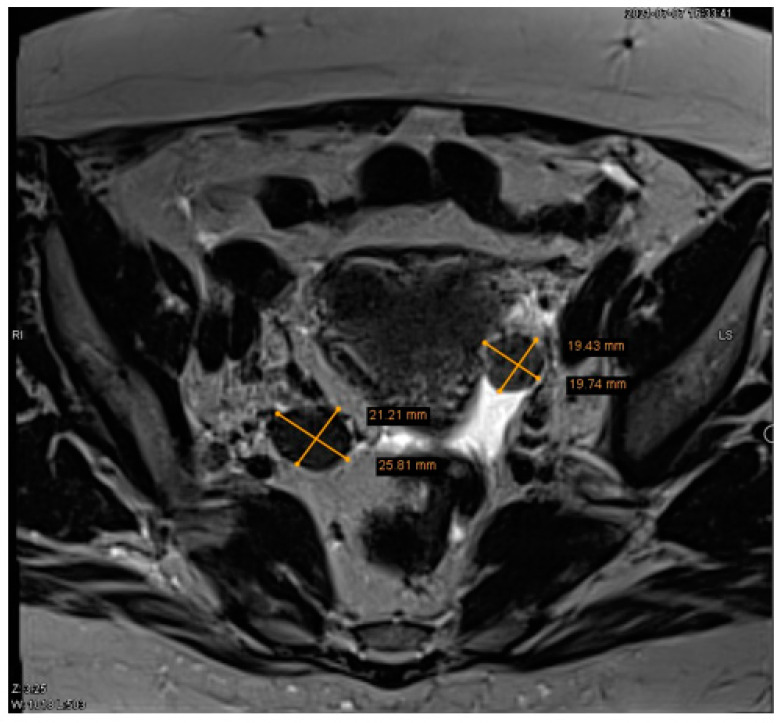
Pelvic magnetic resonance imaging (MRI) of two ovarian lesions. The pelvic MRI revealed a circumscribed left ovarian structure measuring 1.9 × 1.9 cm and a circumscribed right ovarian structure measuring 2.1 × 2.6 cm.
